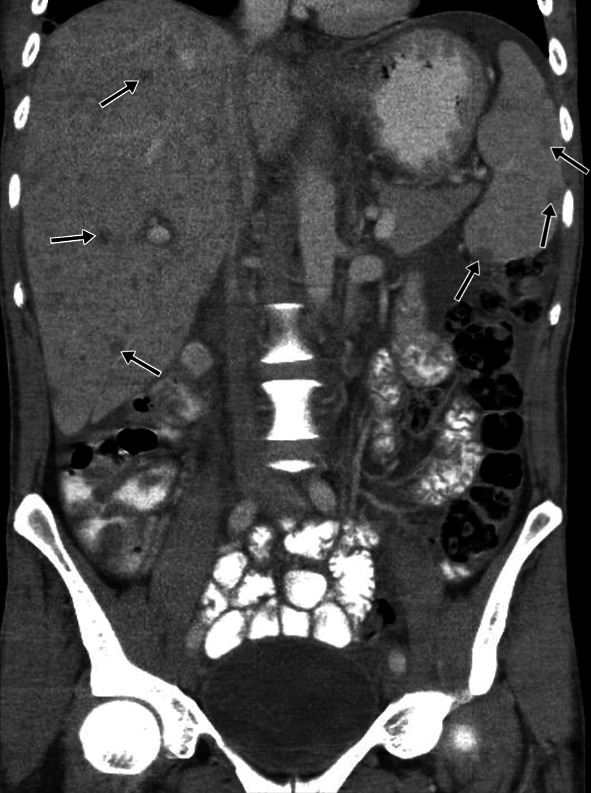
A coronal contrast-enhanced CT image of a 43-year-old male shows multiple hypodense lesions (arrows) in the liver and spleen. Hepatic and splenic TB
